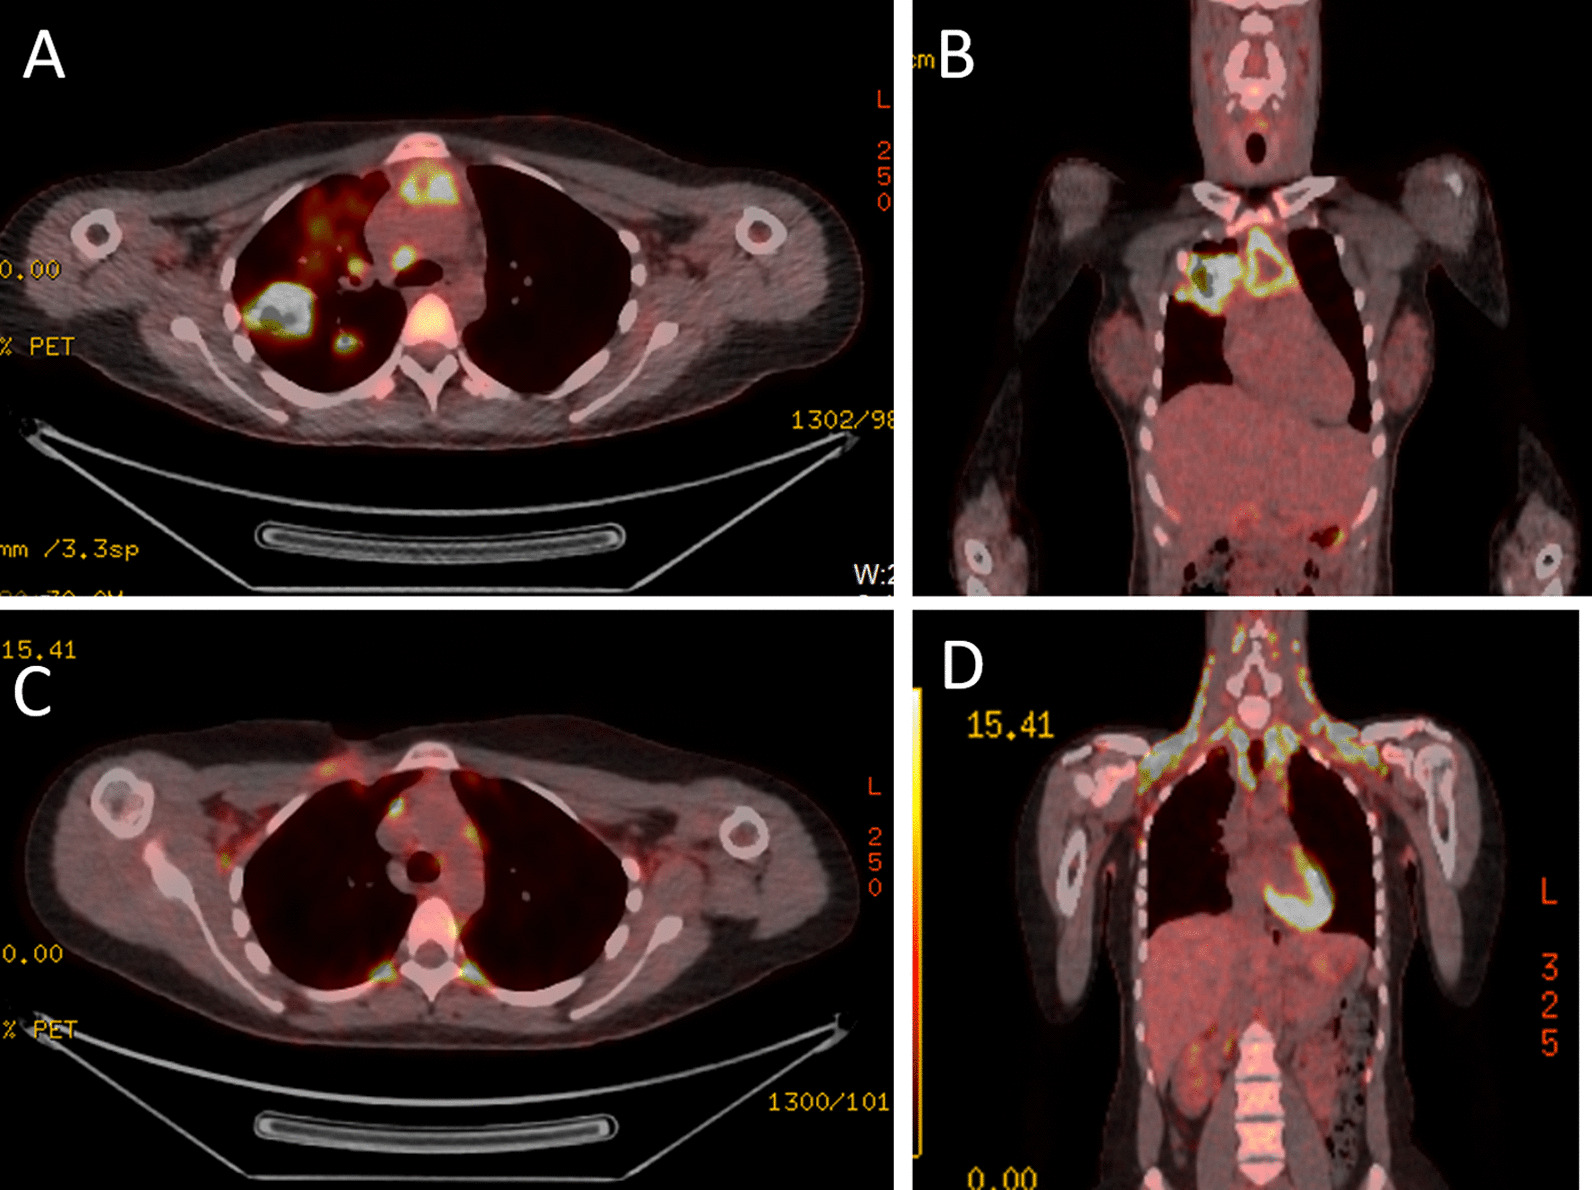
FDG-PET-CT scan images pre-and post-chemotherapy. A Pre-treatment axial section of FDG-PET-CT at mid-thoracic level showing metabolically active necrotic mediastinal lymph nodes and parenchymal lesions in the lungs. B Pre-treatment coronal section demonstrating metabolically active necrotic mediastinal and lung lesions. C Post-treatment axial images of FDG-PET-CT at mid-thoracic level showing complete resolution of the previously noted lung lesions with no significant residual activity. Background uptake seen on these images is due to physiological brown fat activity. D Post-treatment coronal section of FDG-PET-CT with no residual metabolically active disease. Supraclavicular fossa shows marked background of physiological brown fat activity
